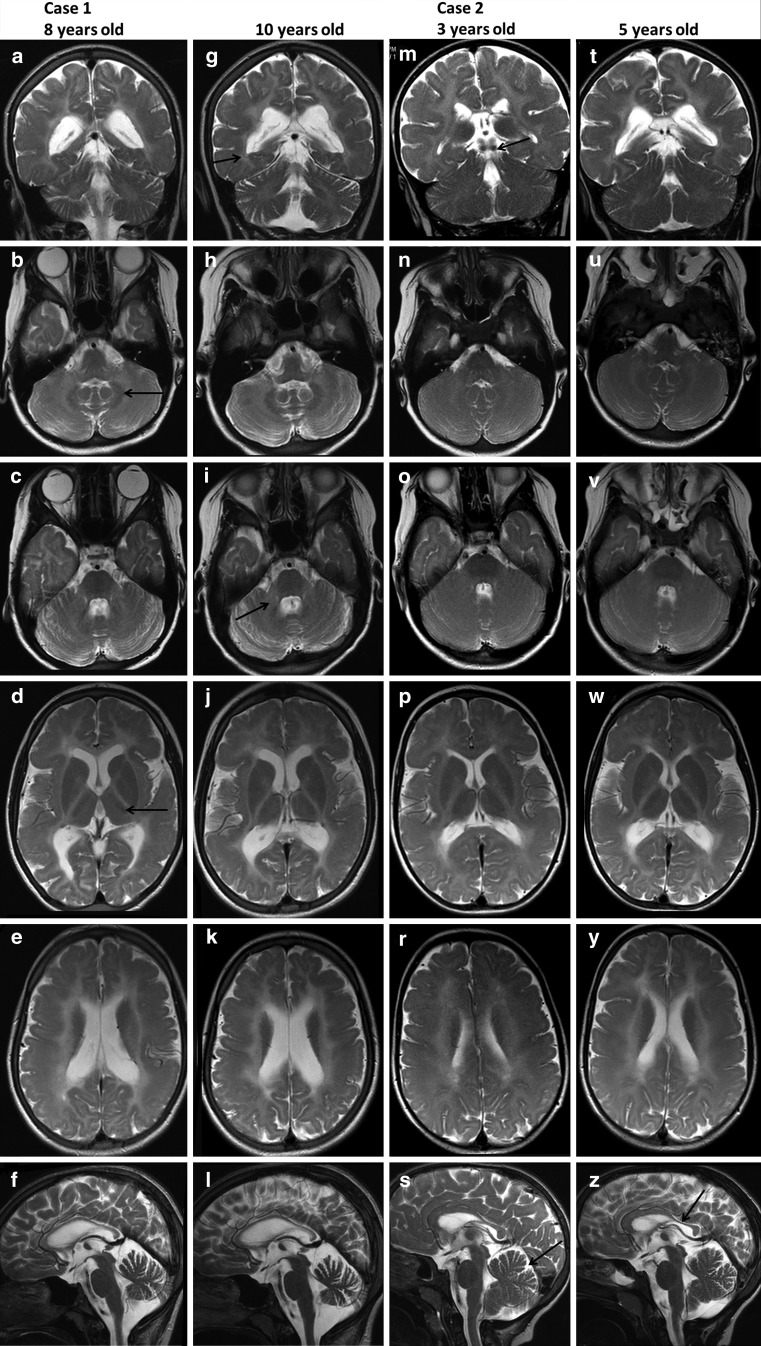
Brain magnetic resonance imaging (MRI) of the patients. T2-weighted images. Case 1 is seen in *A–L*. The first column (*A*–*F*) shows the brain MRI at the age of 8 years while the second column (*G–L*)—the MRI at the age of 10 years. Case 2 is shown in *M–Z*. The third column (*M–S*) shows the brain MRI at the age of 3 years while the fourth column (*T–Z*) shows the MRI—at the age of 5 years. Coronal T2-weighted images demonstrated very thin and slightly myelinated optic radiation (*arrow on G*) and myelinated inferior colliculi (*arrow on M*). Typical hypointensity of the dentate nucleus is seen on axial T2-weighted images (*arrow on B*) more evident in the older girl (*B, H*). Note the hyperintense signal of the middle cerebellar peduncle (*arrow on I*) demonstrating hypomyelination of this structure. In the older girl axial images (*C, I*) mild atrophy of cerebellar hemispheres is seen with little progression over 2 years. Cerebellar hemispheres did not show atrophy in the younger girl (*N, O, U, V*). Diffuse hyperintensity of the cerebral white matter is seen on supratentorial axial images of the brain. Ventroanterolateral nucleus of the thalamus appeared hypointense (*arrow, picture D*). Posterior limbs of internal capsules are not myelinated. Mild enlargement of the lateral ventricles is visible. Corpus callosum is thin and unmyelinated on midline sagittal T2-weighted images of the older girl (*F, L*). Note atrophy of the vermis. Slight progression of the atrophy of the vermis is seen. Corpus callosum of the younger girl (*S, Z*) is also unmyelinated and thinned posteriorly (isthmus and splenium); slight progression of changes. Note prominent, but within normal limits, primary fissure of the vermis (*arrow, picture Z*), which remained unchanged over 2 years
